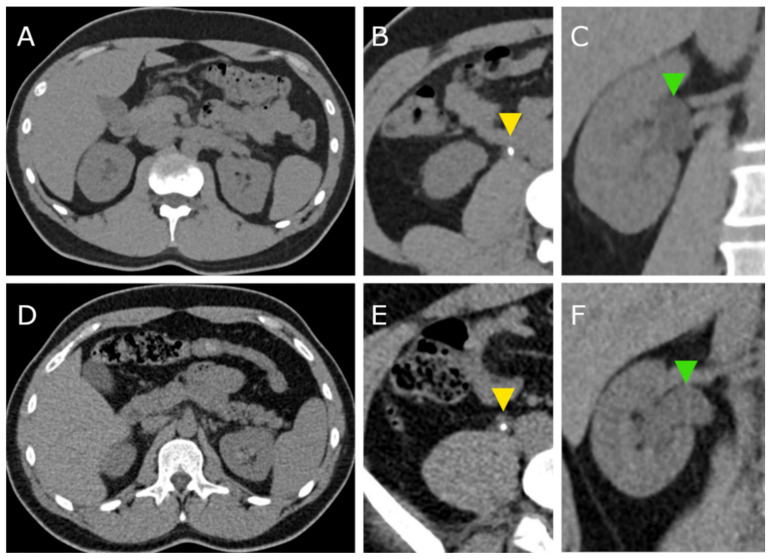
CT images acquired with the photon counting CT (PCCT, A–C) and with the standard CT (S40, D–E). Yellow arrowheads (B,E) indicate ureter stones in both patients. Green arrowheads (C,F) point to the dilated renal pelvis due to the ureter obstruction.
